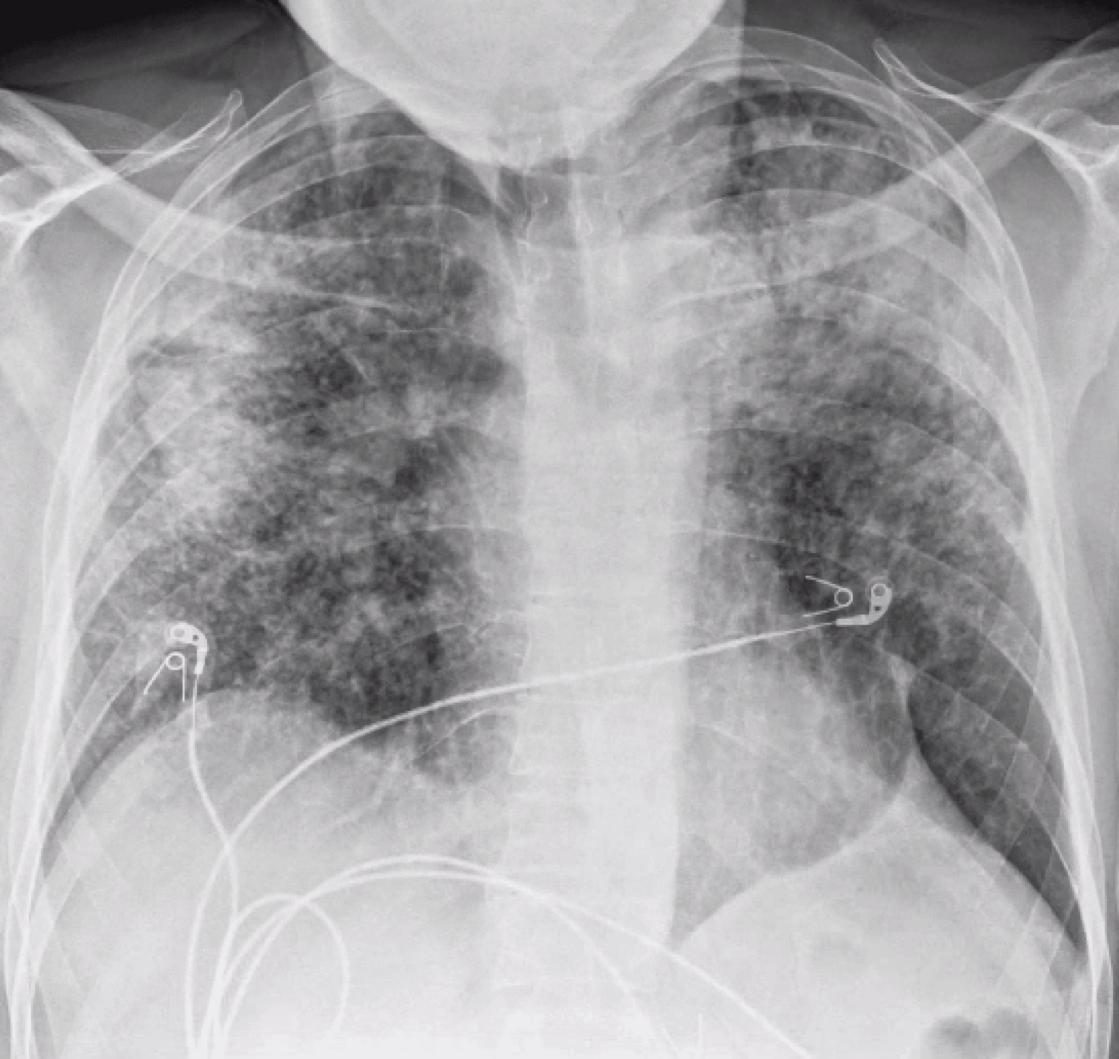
CXR at admission CXR, chest radiograph
